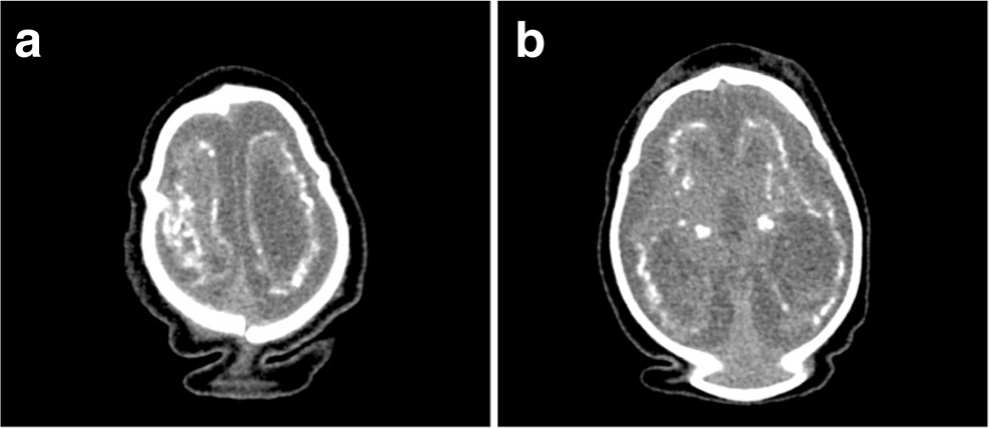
a Overriding of the cranial bones, cerebral atrophy, and anarchic distributed coarse calcifications; the excess skin as a result of the decrease in skull volume. b CT scan with gross calcifications in the basal ganglia and subcortical cortical transition
